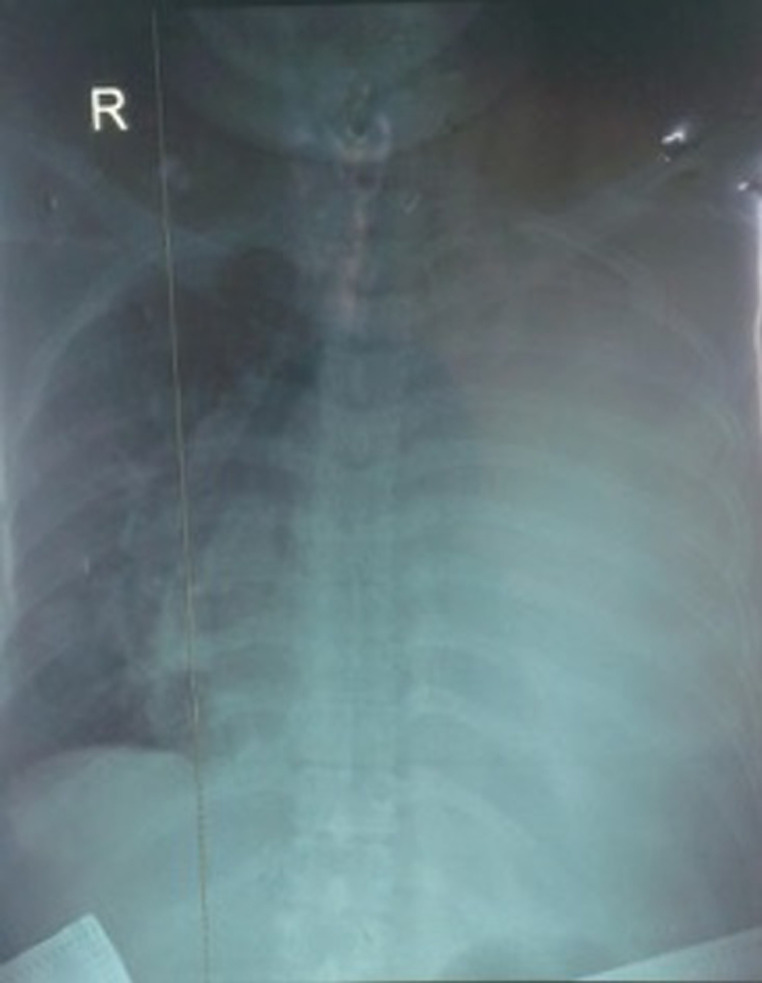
radiographie du thorax de la patiente
